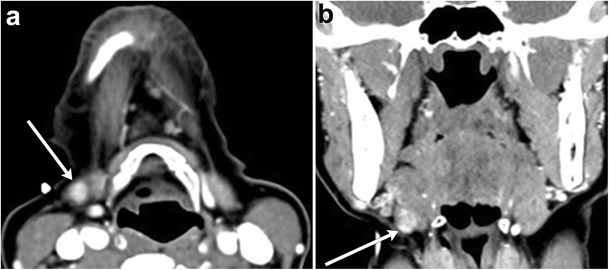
Pleomorphic adenoma of the submandibular gland. a Axial and b coronal contrast-enhanced CT images demonstrate an enhancing well-circumscribed round mass within the inferior aspect of the right submandibular gland (*arrow*)
